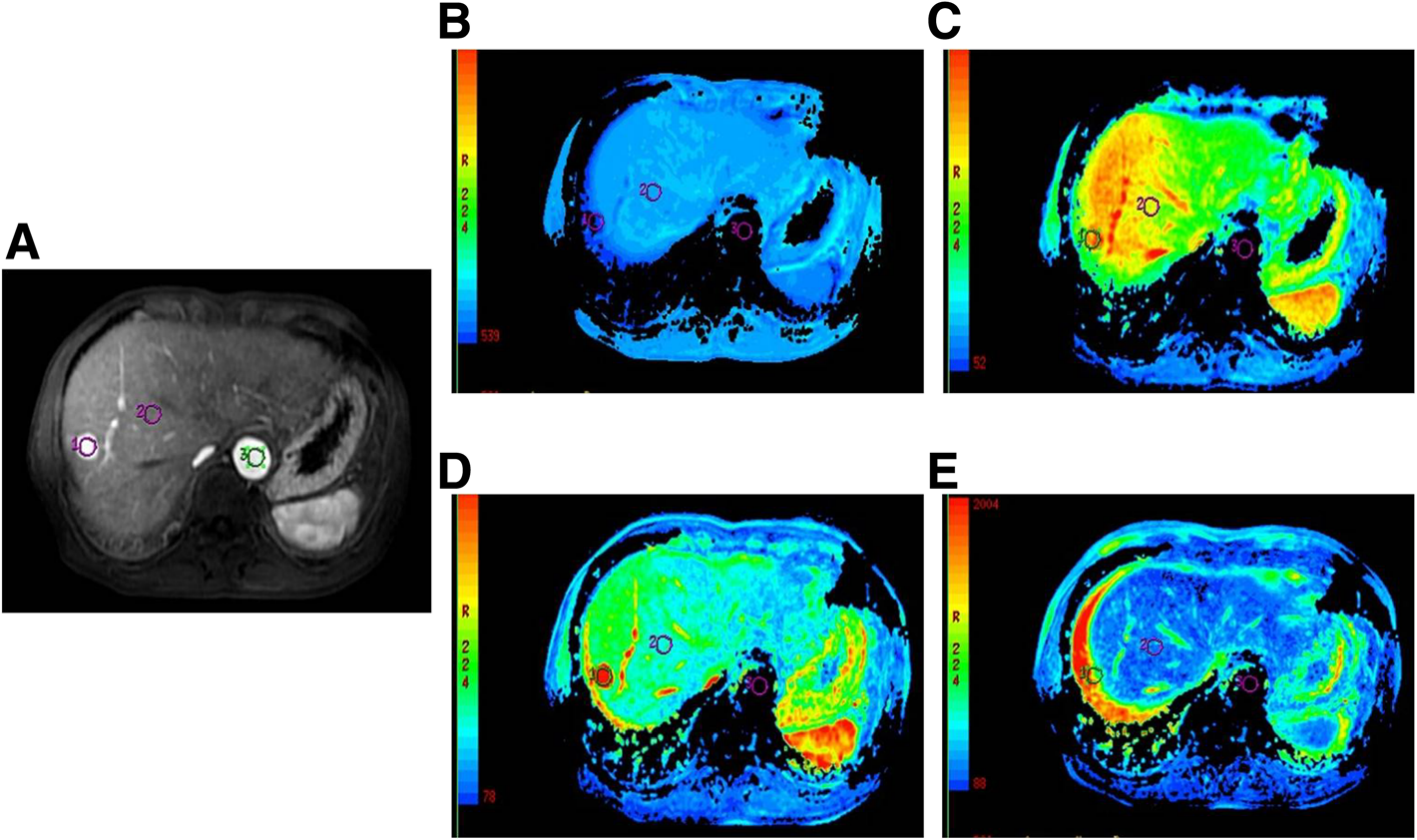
Imaging from a patient of male, 59 years old with high differentiation HCC. Figs. a-e represent dynamic contrast-enhanced magnetic resonance imaging and the pseudo-color images of MET, PET, MSI, and MSD, respectively
